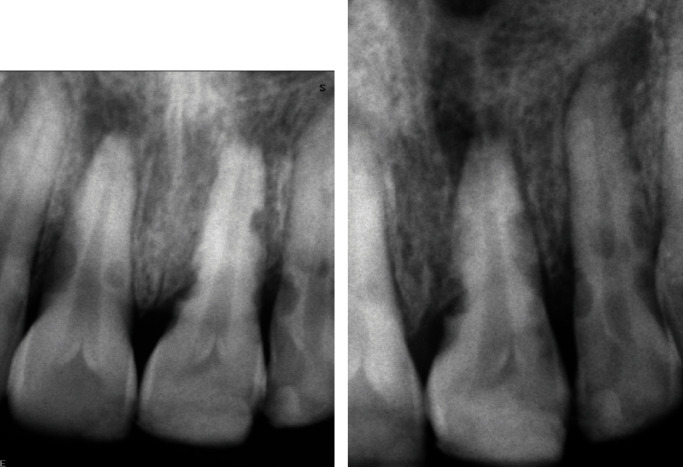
Pre-operative radiographs showing multiple external inflammatory root resorption lesions. (a) Teeth number 11 and 21. (b) Teeth number 21 and 22.
